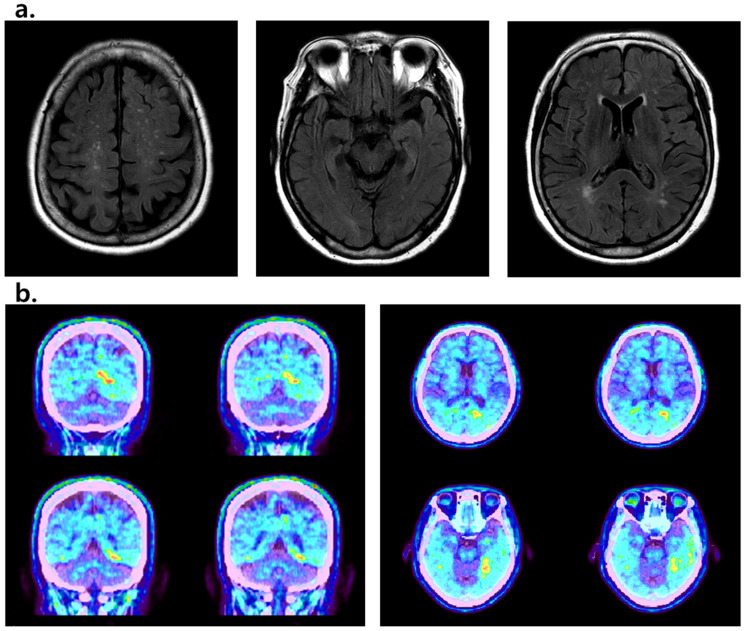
(a) MRI data of the proband patient, which showed diffuse cortical atrophy and enlarged ventricles; (b) PET-CT data from the patient showed amyloid deposits in different brain areas.
